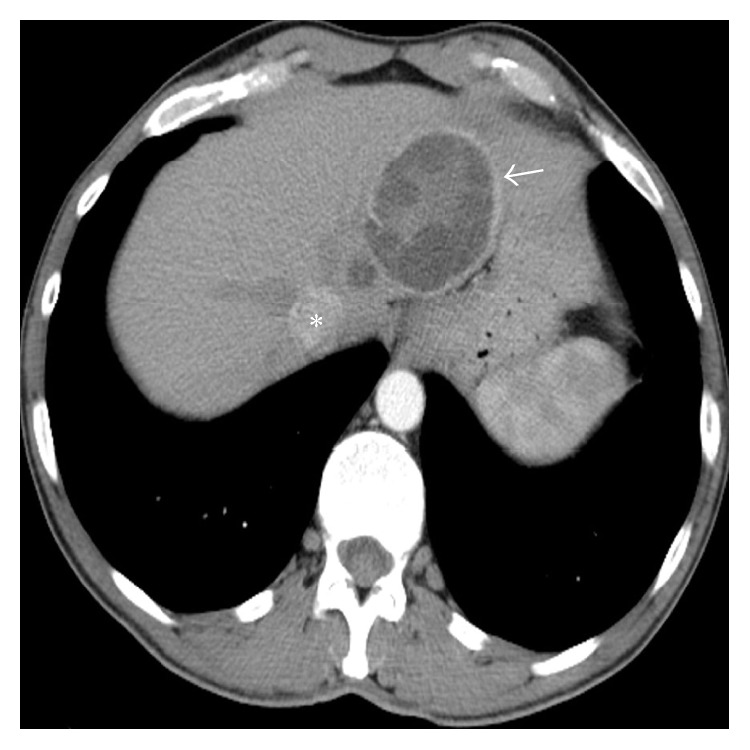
Contrast-enhanced CT scan reveals typically hydatid liver cyst as CE 3a cyst following the WHO classification (arrow) adjacent to the IVC (asterisk).
